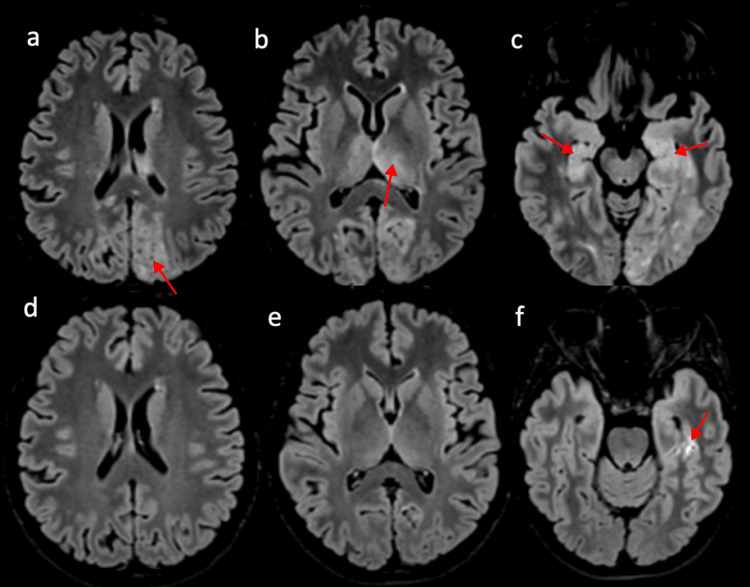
Axial flair images at the onset ( a,b,c) and two months later (d,e,f). The initial FLAIR images show hyperintense cortico-subcortical edema in the parietal, occipital and temporal lobes, with the most severe involvement affecting the left temporal lobe. There is also central involvement with thalamic and posterior callosal hypersignal. The memory impairment is likely correlated with the involvement of both hippocampi (image c, solid arrows). FLAIR images taken two months later showed resolution of all hyperintensities, except in the left inferior temporal cortex, indicating chronic ischemia (image f). FLAIR: Fluid-Attenuated Inversion-Recovery
